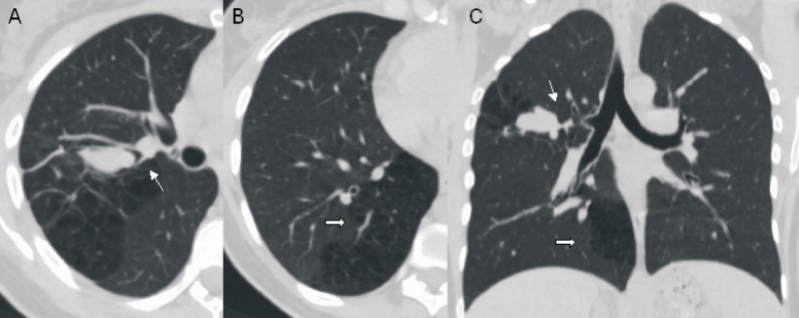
Multi-detector computed tomography scan. (a) Axial image shows a mucus-like density opacity at the right upper lobe. The bronchial branch for the right upper lobe is not identifiable. (b) Axial image of the right lower lobe allows the characterization of a malformed multicystic area of the lung parenchyma. (c) Malformative features in minimum intensity projection (minIP) coronal reconstruction (bronchial atresia: thin arrow; congenital cystic adenomatoid malformation: thick arrow).
